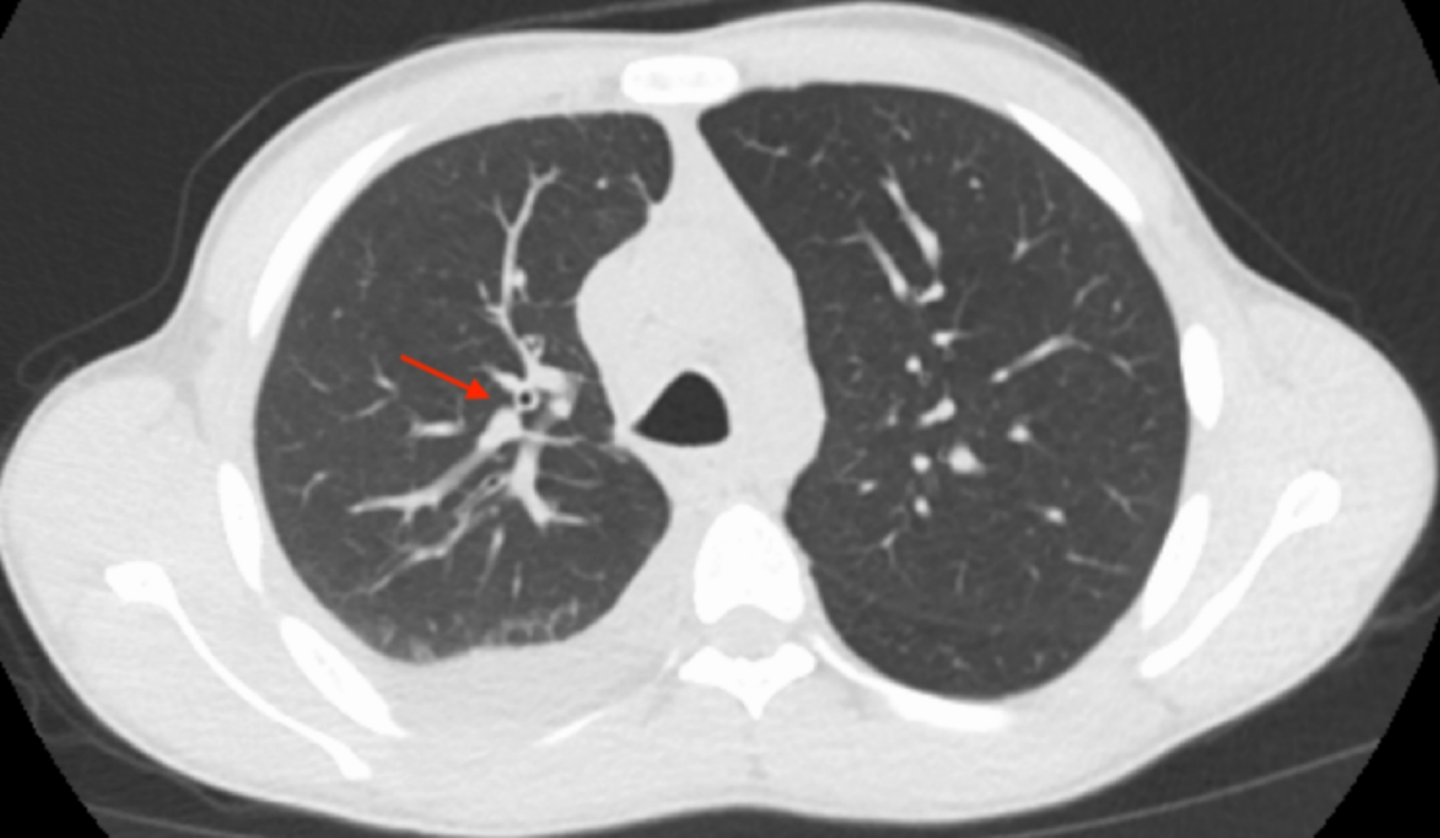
CT of the thorax demonstrating right middle lobe tree-in-bud nodularity and bronchiectasis (red arrow).
